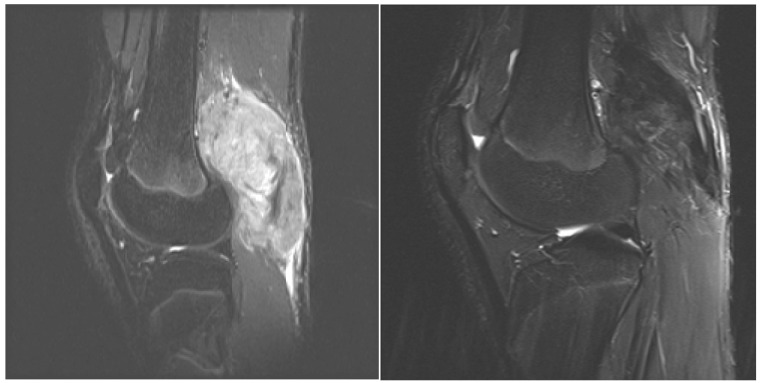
T2 sagittal MRI images before and after treatment demonstrate significant reduction in lesion size after treatment.
